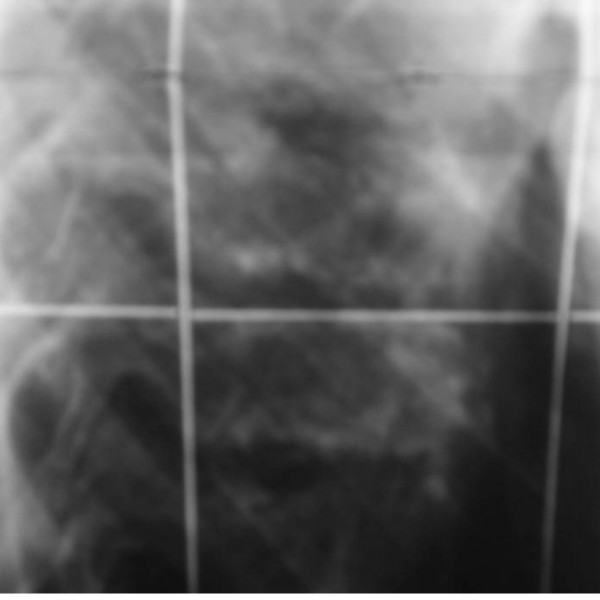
Lateral spine radiograph showed severe lytic changes with extensive irregularities of the anterior/posterior end plates.
